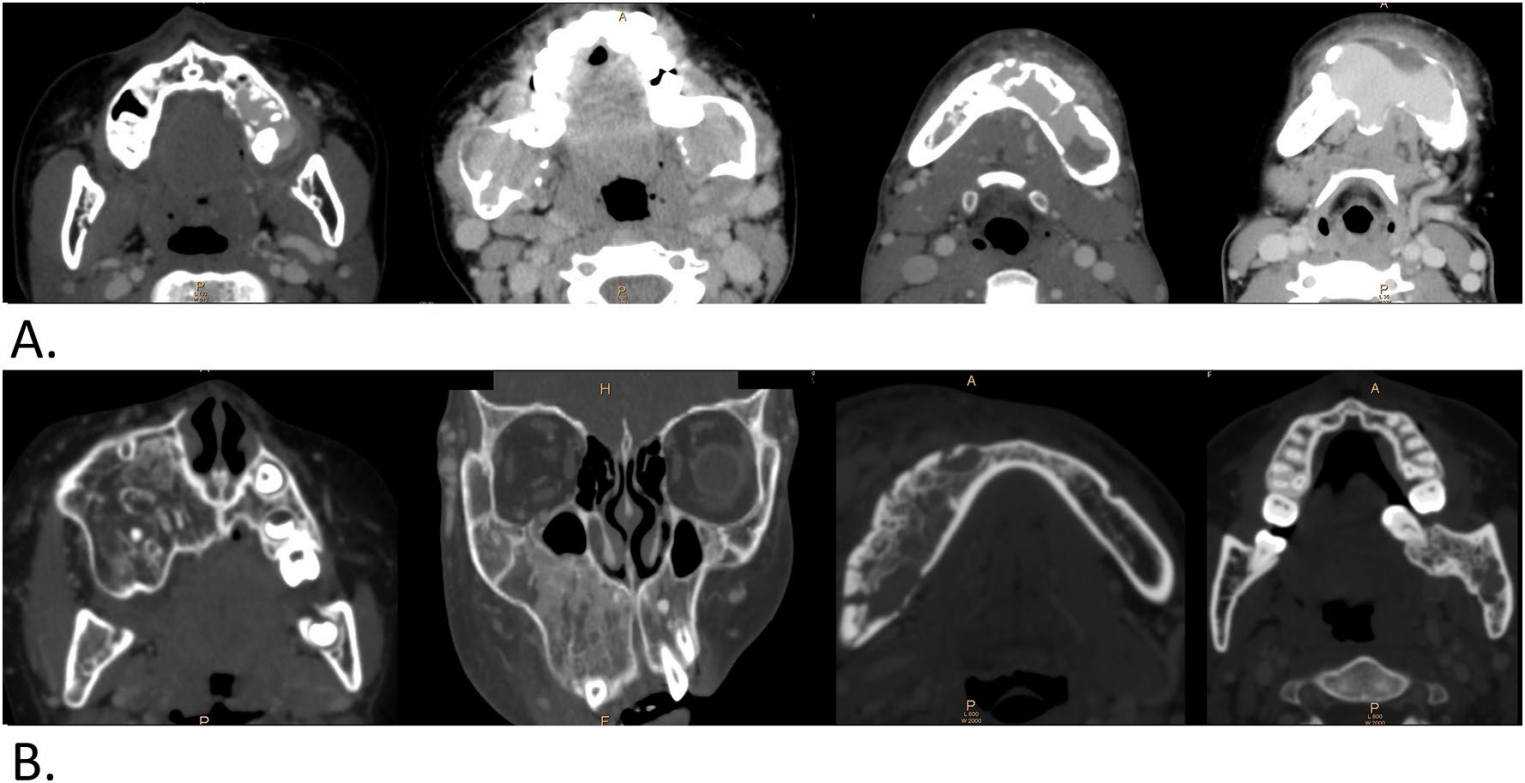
Enhancement in the cancellous bone on contrast-enhanced CT and manifestations of j-AVMs. Enhancement (a) centred on the root of the left first molar in the maxilla; the cancellous bone of the bilateral mandibular ramus; the anterior and posterior region of the mandible on the left side; the anterior region of the mandible with destruction and penetration of the cortical bone. (b) Irregular hyperosteogeny, with disordered and heterogeneous bone density and structure on contrast-enhanced CT and manifestations of j-AVMs. Irregular hyperosteogeny involves the whole maxilla of the left side; buccal cortex of the right mandible; area around the root of the teeth.
